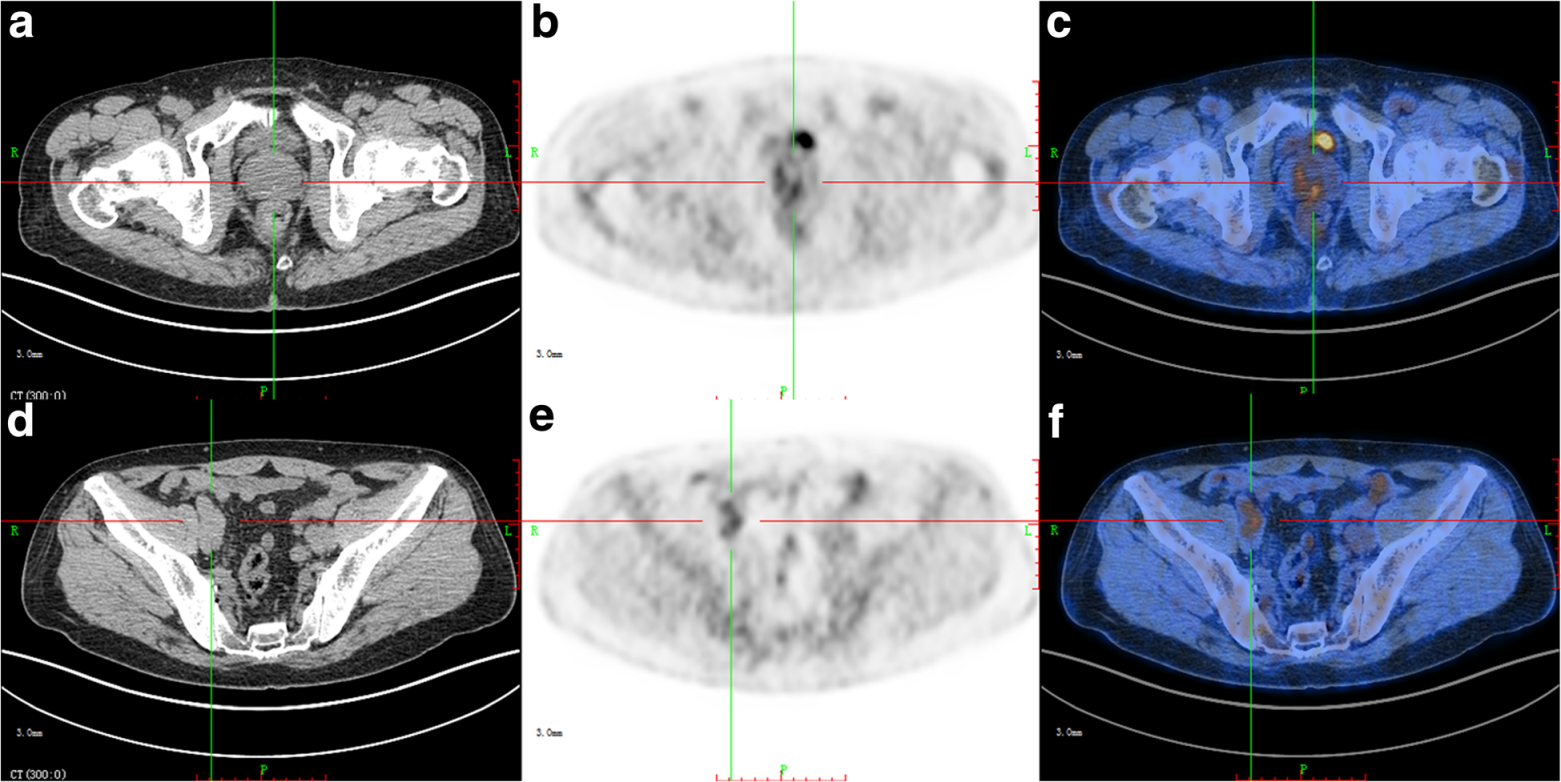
Positron emission tomography/computed tomography (PET/CT) scan of pelvic cavity of the second patient. a. Non-contrast CT scan of the prostate, b. PET scan of the prostate, c. Fused PET/CT scan of the prostate, d. Non-contrast CT scan of the lymph nodes in the pelvic cavity, e. PET scan of the lymph nodes in the pelvic cavity, f. Fused PET/CT scan of the lymph nodes in the pelvic cavity
